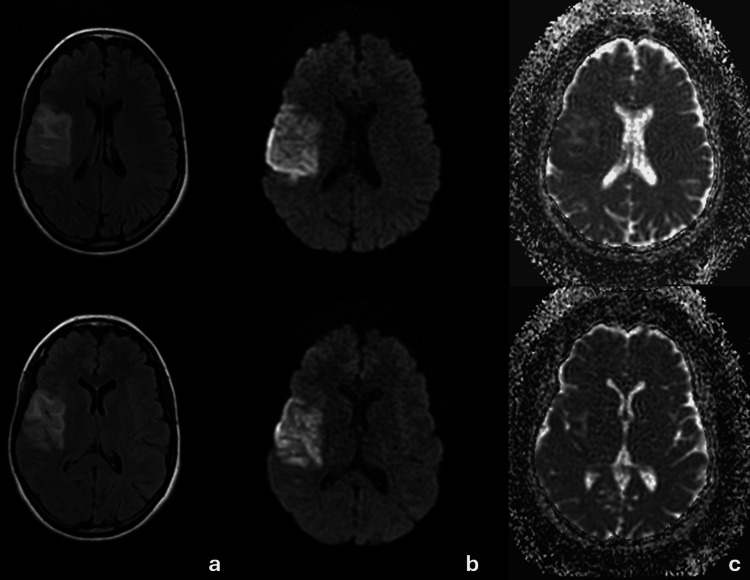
Brain MRI showing a cortico-subcortical infarct in the right fronto-parieto-insular region. (a) FLAIR sequence demonstrating early hyperintensity; (b) diffusion-weighted imaging showing hyperintensity; (c) apparent diffusion coefficient map demonstrating low signal, consistent with subacute ischemic infarction in the superficial territory of the right middle cerebral artery.
